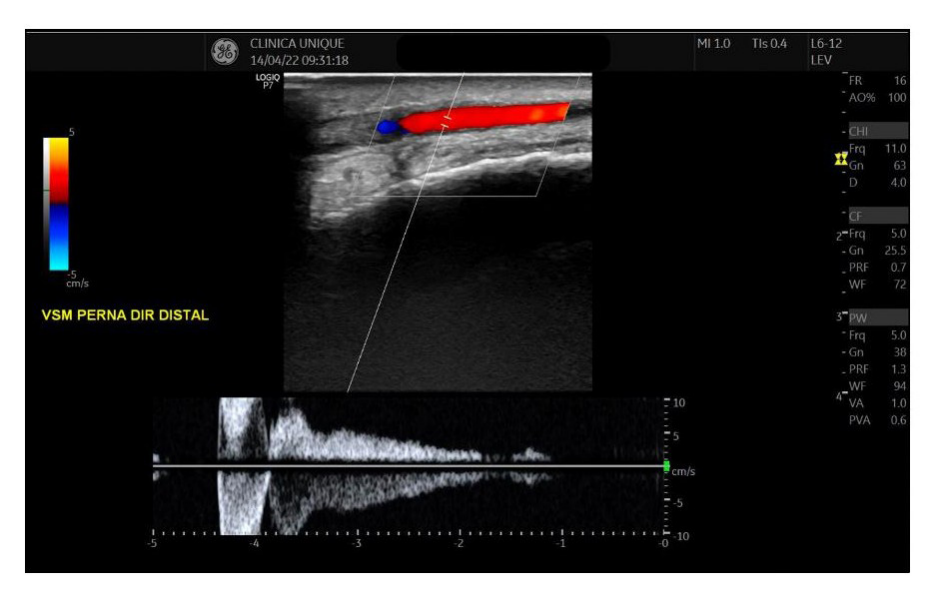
Duplex ultrasound image from the initial consultation demonstrating significant reflux in the great saphenous vein. GSV: Great saphenous vein
